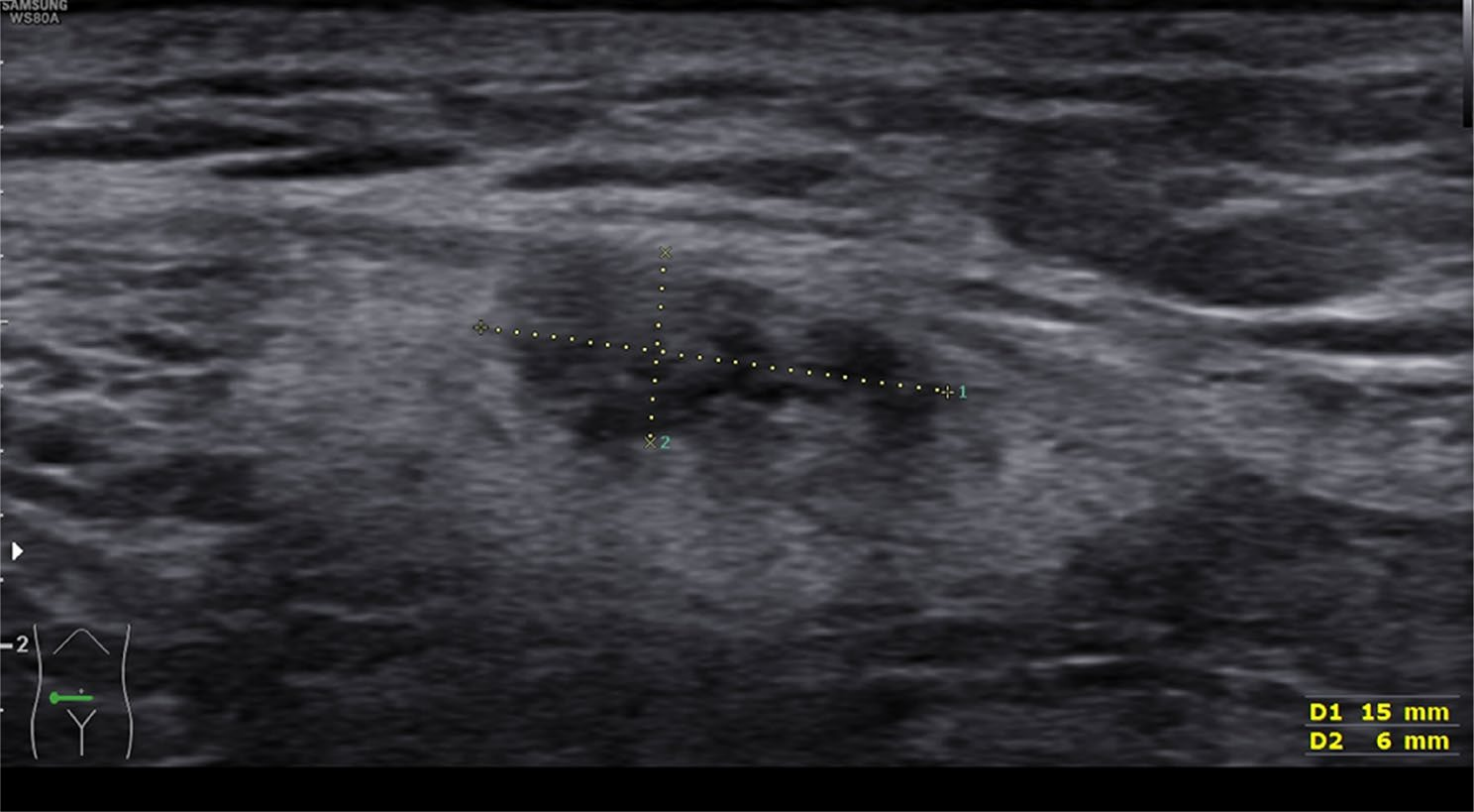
Transabdominal ultrasound to identify a deep endometriosis nodule in the abdominal wall (#Enzian(u) FOabd. wall)
